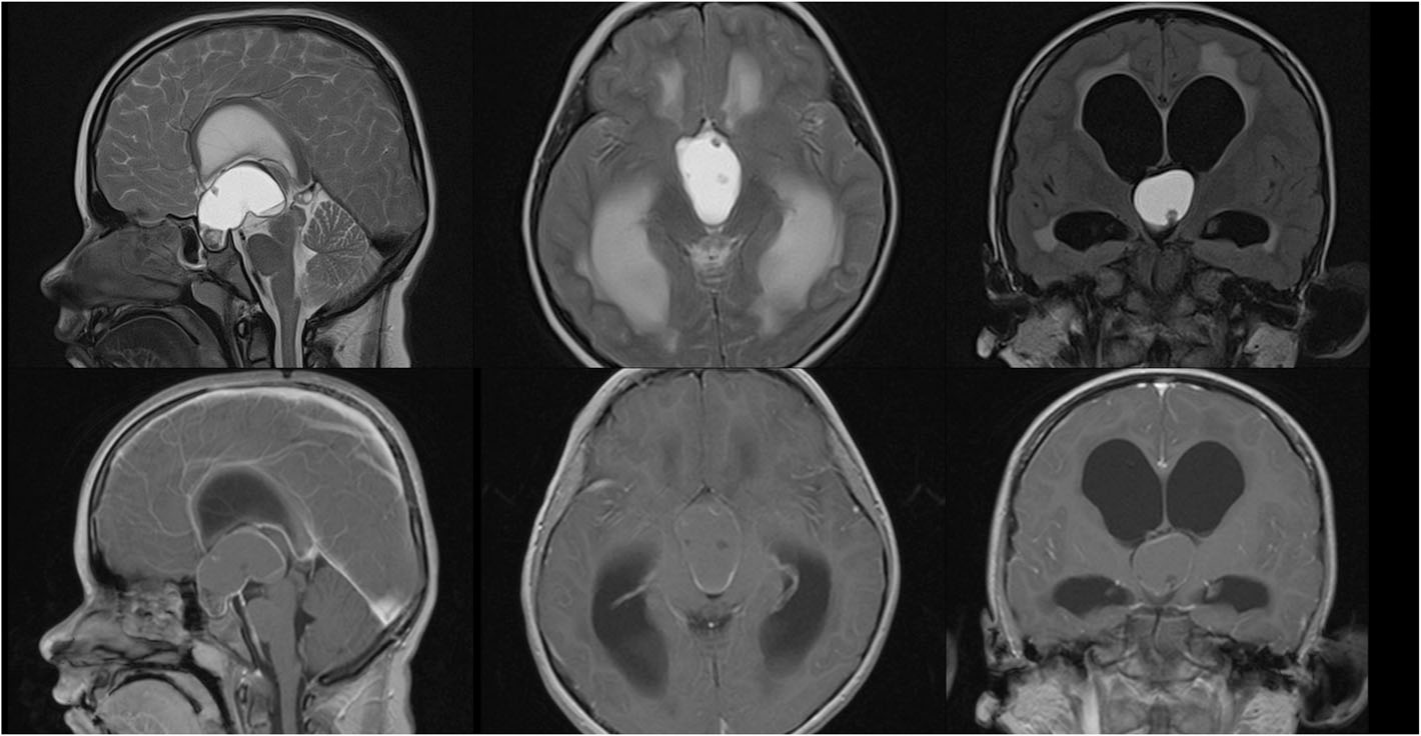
Preoperative magnetic resonance imaging scan (17 June 2016). The pathological area with fluid accumulation (APxCCxLR: 45x35x27 mm) in sellar and suprasellar region. Tumor tissue well demarcated. The suspicion of proliferative process, craniopharyngioma. Supratentorial part of the ventricular system shows characteristic features of hydrocephalus with transependymal oedema
